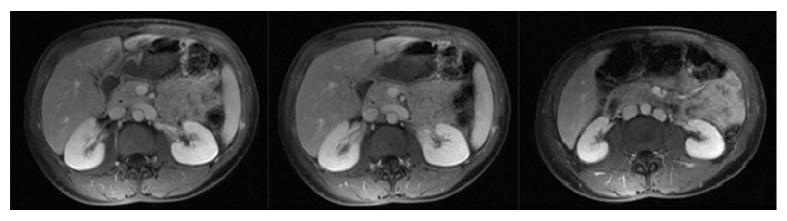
An outpatient MRI of the abdomen shows no hydronephrosis at the time of no symptoms. Incidentally noted is a duplicated right inferior vena cava distal to the renal vein.
